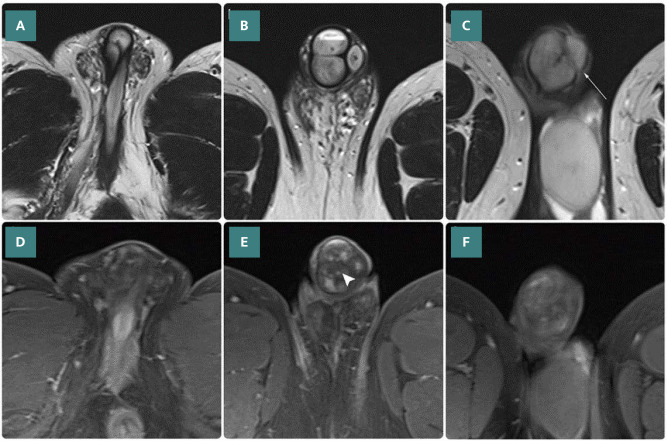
Axial diffusion-weighted MRI scan of the penis. A. Diffusion-weighted sequence, at a high b value of 1,000, shows a small focus of high-signal intensity at the left corpus cavernosum at the body level (arrow). B. Apparent diffusion coefficient map shows the corresponding focus with a lower apparent diffusion coefficient in the same region of interest, reflecting an element of reduced perfusion.
